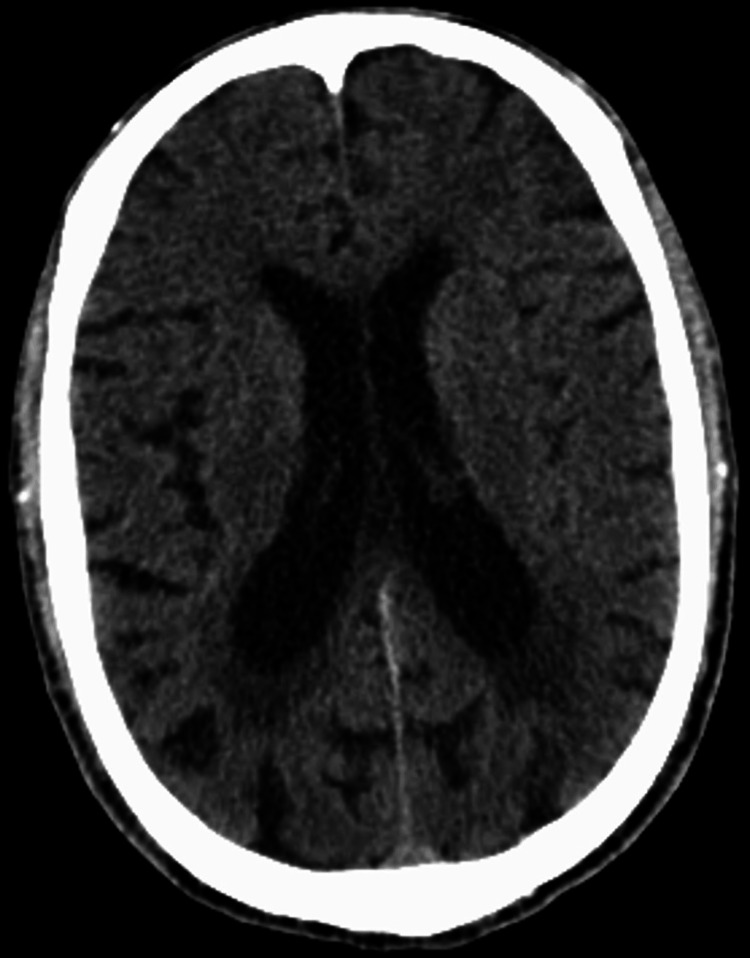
Non-contrast CT of the brain with no acute intracranial abnormality, intracranial hemorrhage, or mass effect. Age-appropriate volume loss and moderate white matter small vessel ischemic changes can be noted.
